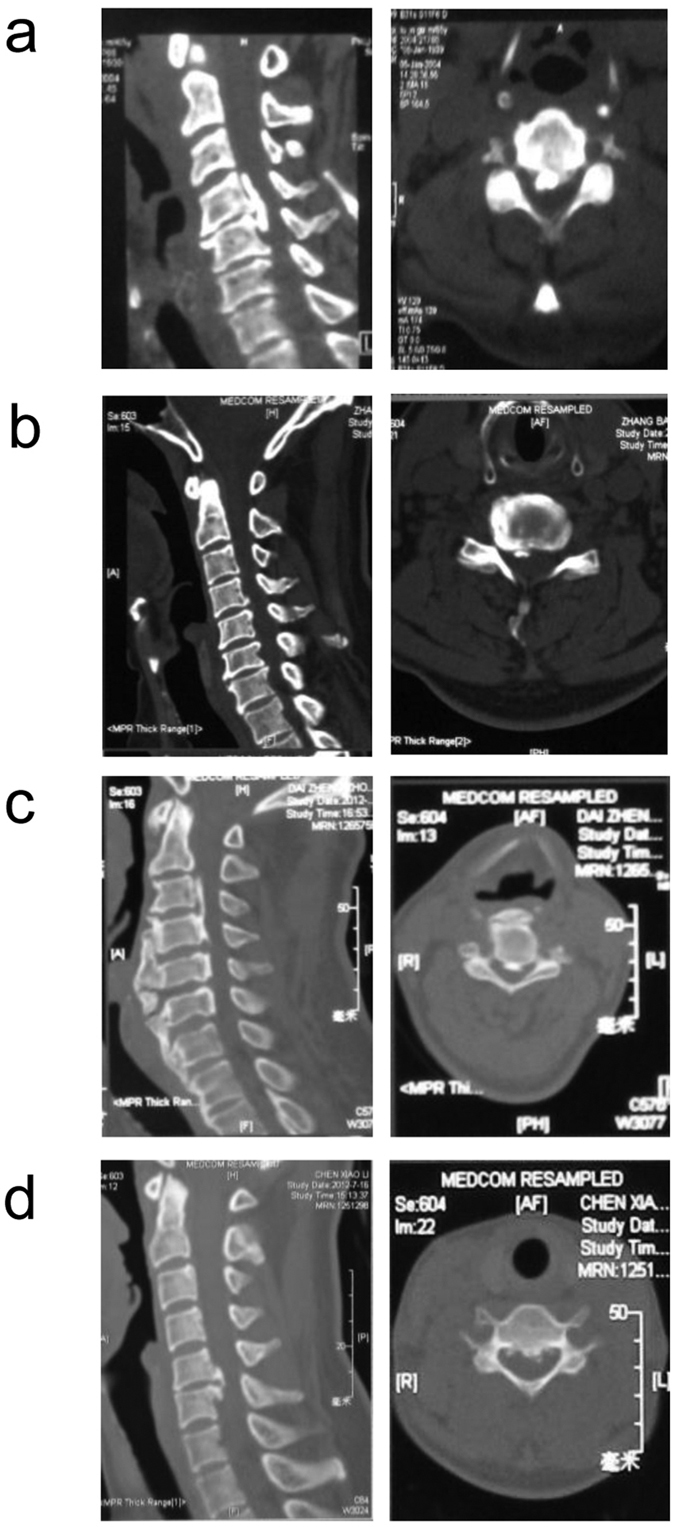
Radiological analysis of cervical spine in four subtypes of OPLL. (a) The Continue subtype OPLL at C4-C5 and cervical stenosis are observed in subject R0898 with c.2260C > T in *FGFR1*. (b) The Segmental subtype of OPLL at C4-C6 and cervical stenosis are found in subject R0865 with c.233G > A in *FGFR1*. (c) The Mixed subtype of OPLL at C3-C6 and cervical stenosis are seen in subject R0843 with c.1144G > A in *COL6A1*. (d) Also, the Local subtype of OPLL at C5 and C6 is observed in subject R0875 with c.2953G > A in *COL6A1*. Left panels: sagittal MRI; Right panels: axial CT.
